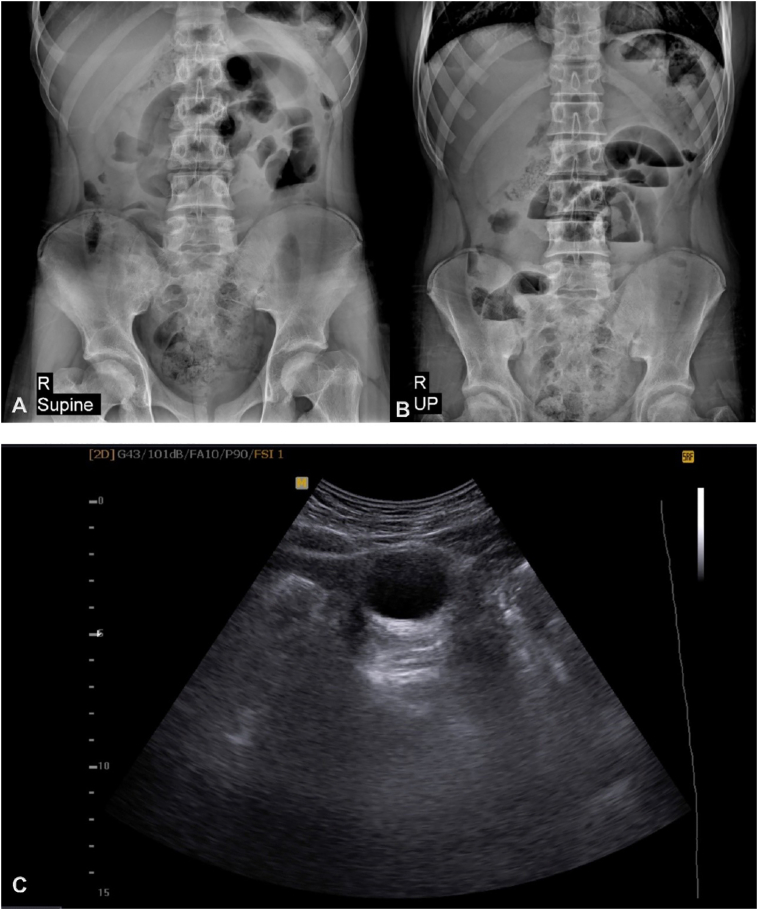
Abdominal X-rays, supine (A) and upright (B), demonstrate distended small bowel loops in mid-abdomen with air-fluid levels in favor of small bowel obstruction. Ultrasound image (C) shows a cystic structure underneath the abdominal wall.
